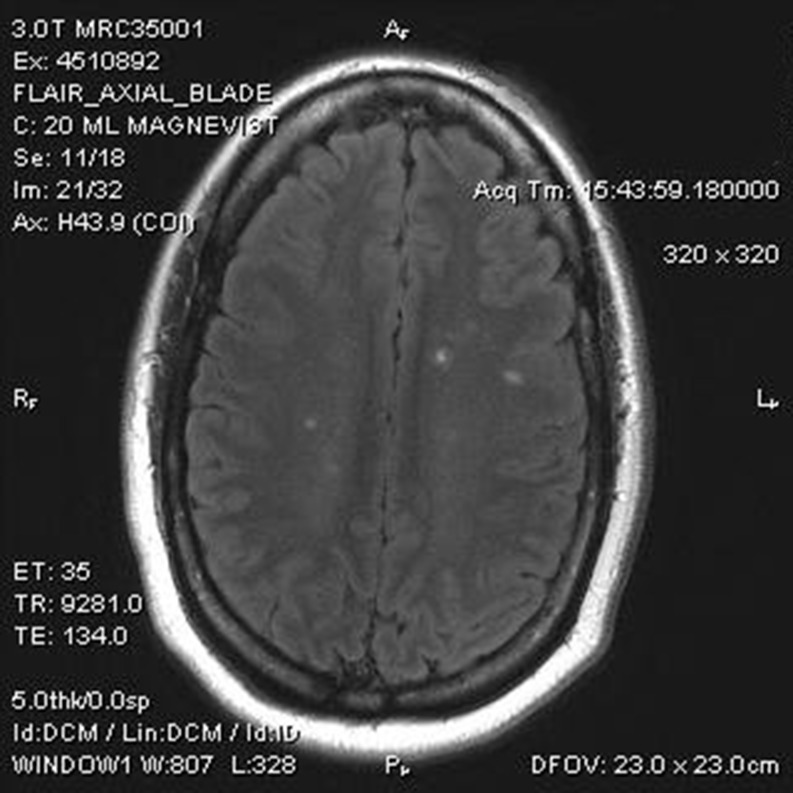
Brain magnetic resonance imaging T2/FLAIR image
